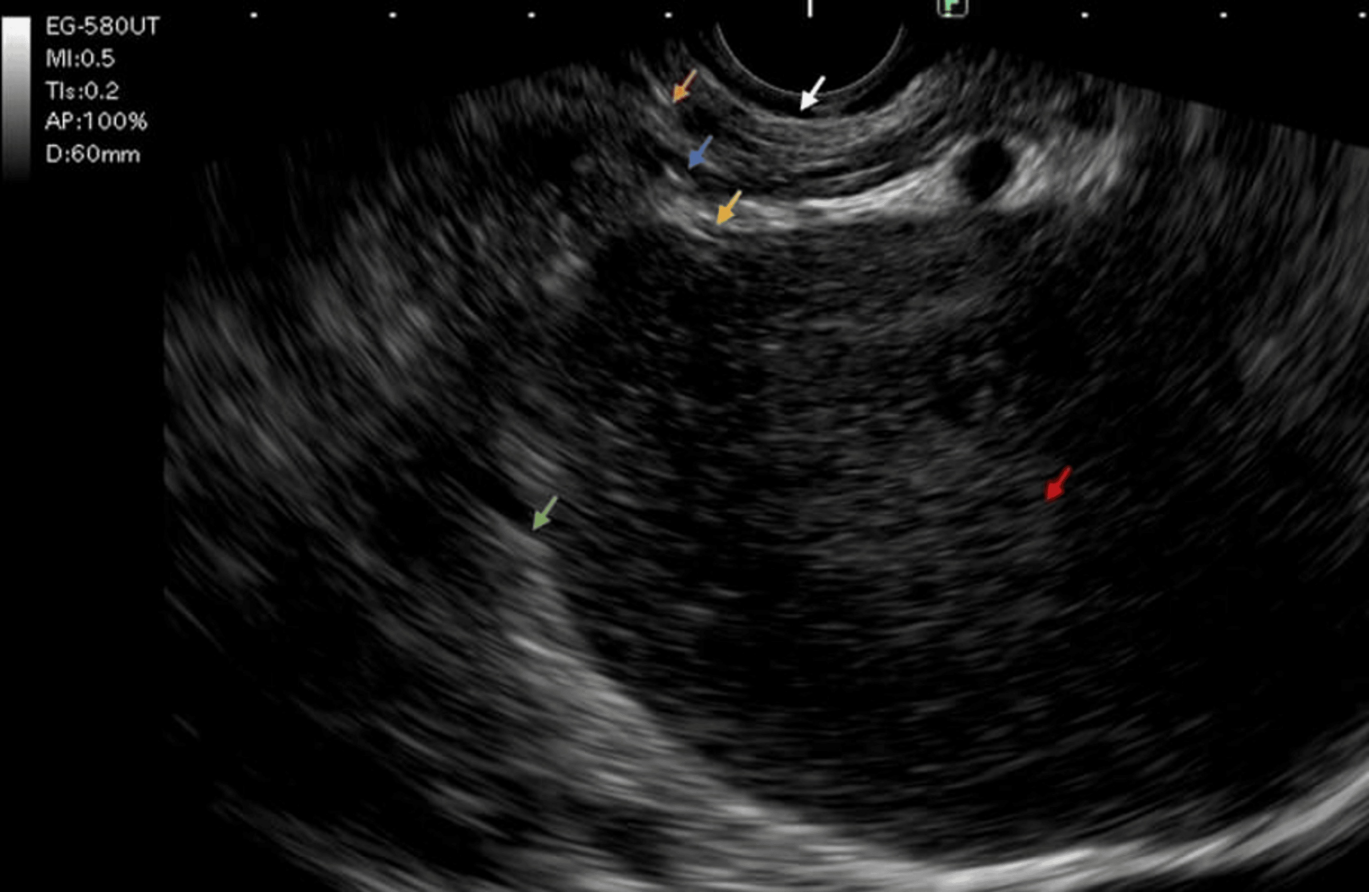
Endoscopic ultrasound (EUS) image showing the cystic lesion in relation to the stomach (pseudocyst of the pancreas/gastric GIST) White arrow: Superficial mucosal layer of the stomach, Orange arrow: Deep mucosa of the stomach, Blue arrow: Submucosal layer of the stomach, Yellow arrow: Muscularis propria of the stomach, Green arrow: Serosa of the stomach, Red arrow: a cystic lesion of size 10*12*10 cm with a wall thickness of 7 mm without any septations. The lesion appears to be related only to the serosal layer of the stomach.
